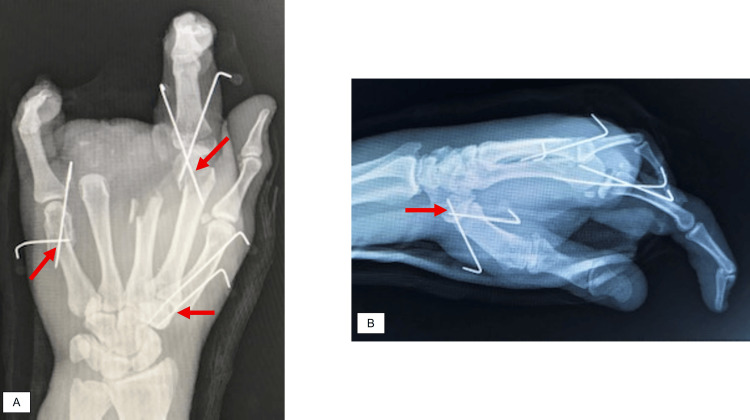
Postoperative radiographs after skeletal fixation An anteroposterior view (A) and lateral view (B) of the left hand demonstrate skeletal fixation of the second and fifth metacarpal fractures as well as the first CMC joint (red arrows). CMC, carpometacarpal
